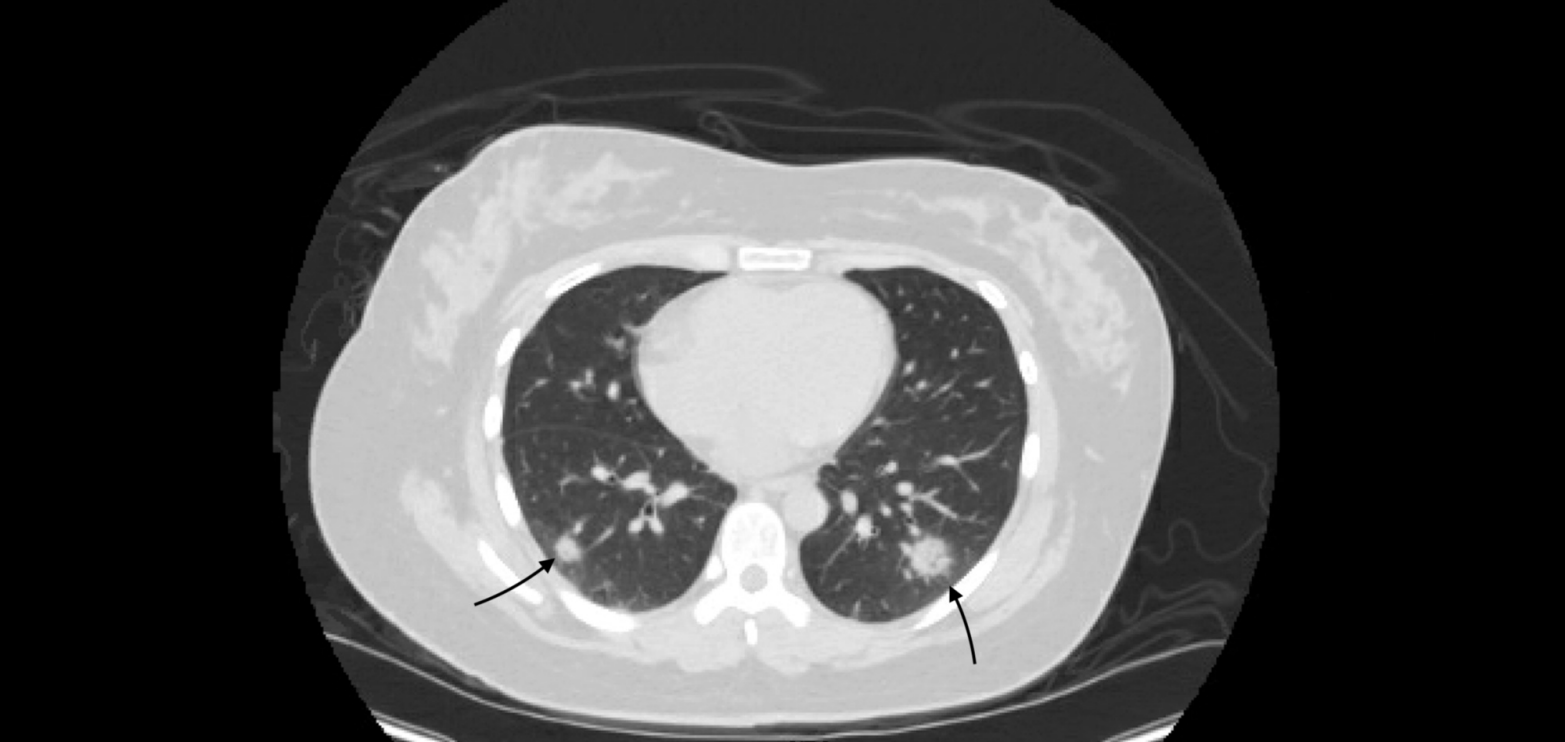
CT of the abdomen and pelvis without contrast revealing multiple cavitating pulmonary nodules
